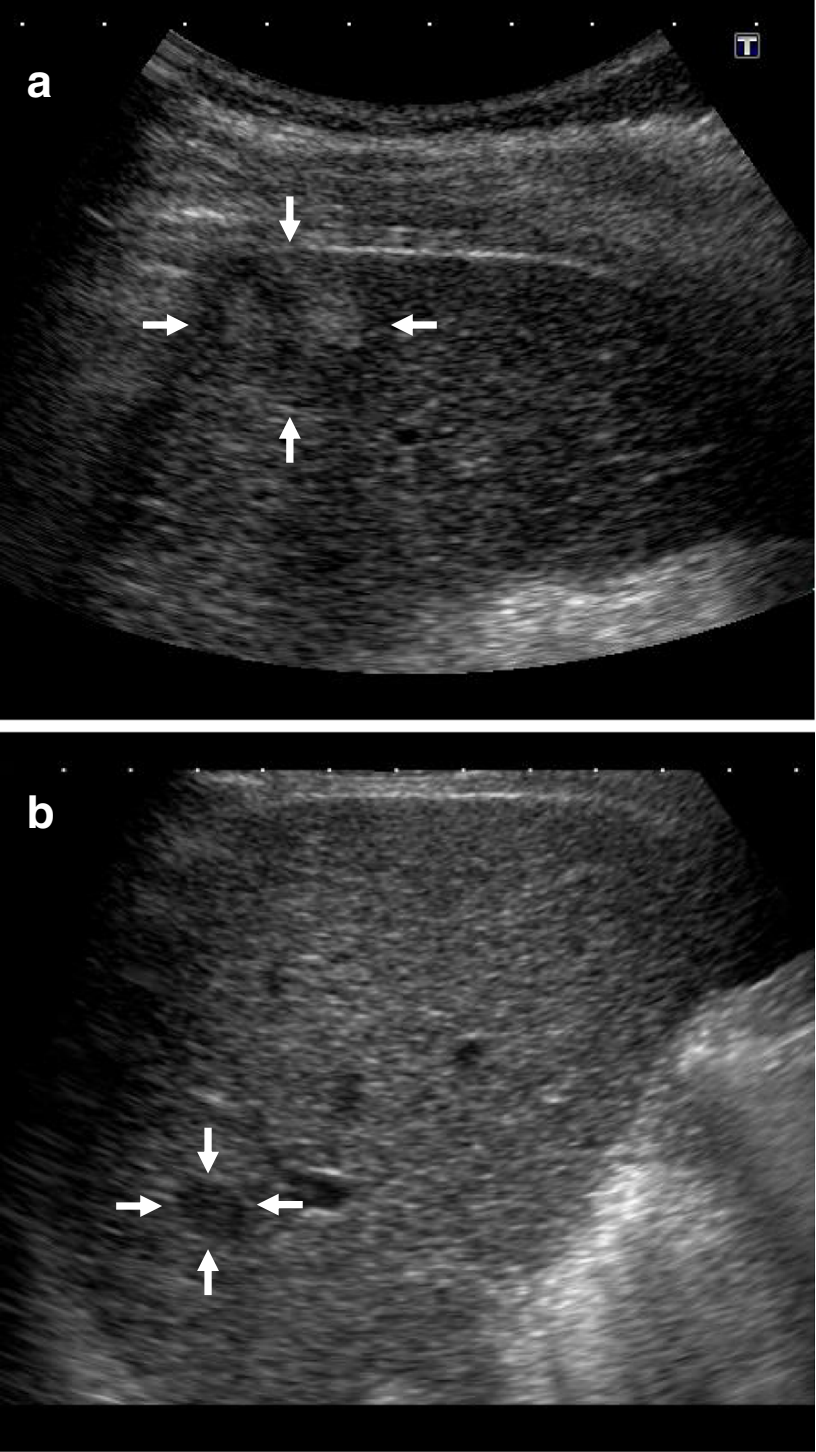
Abdominal ultrasonography (US) showed the two tumors. a A well-defined 23-mm hypoechoic mass that was heterogeneous on the inside was observed in the 6^th^ segment of the liver (*arrows*). b A slightly ill-defined 10-mm hypoechoic mass that was homogeneous on the inside was observed in the 7^th^ segment of the liver (*arrows*)
